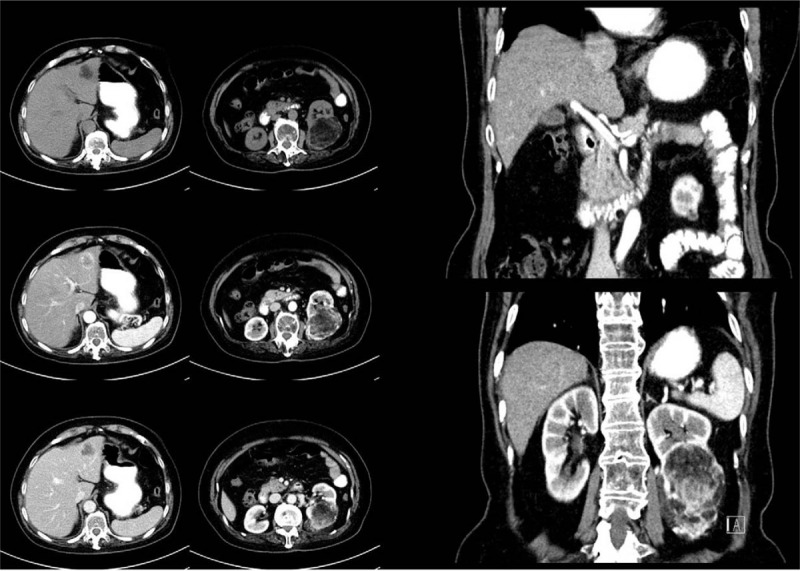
The computed tomography (CT) examination revealed a lobulated low-density soft tissue mass in the left liver, showing a ring-shaped enhancement at the edge of the mass in the enhanced scanning arterial phase and a lower enhanced density than the surrounding liver tissue in the venous phase and the parenchymal phase. And under the left kidney, an irregular mixed density tumor was found, which contained fat density, flocculent soft tissue density, and calcified nodules. The soft tissue was obviously strengthened during the enhanced scanning, and large vessels were visible at the margin and inside of the tumor.
